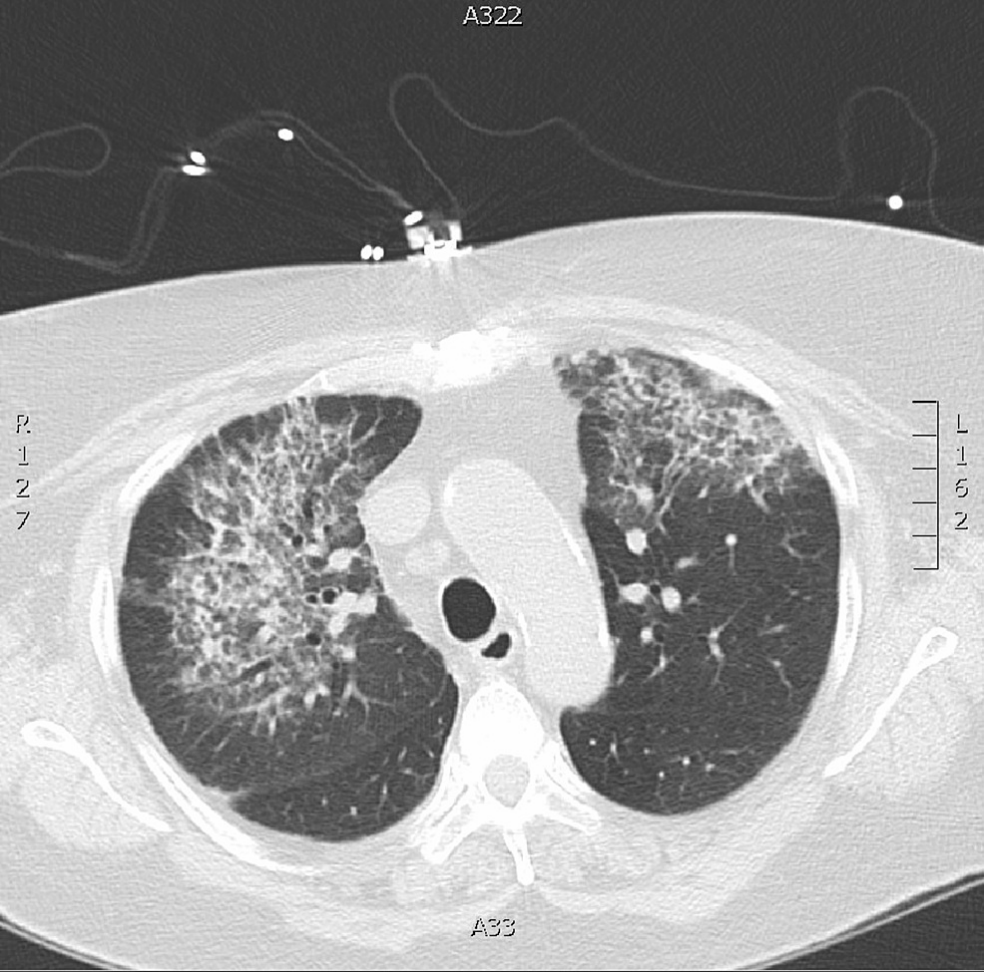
Chest computed tomography scan (lung window) at eight months post-original discharge showing bilateral infiltrates and ground glass.
